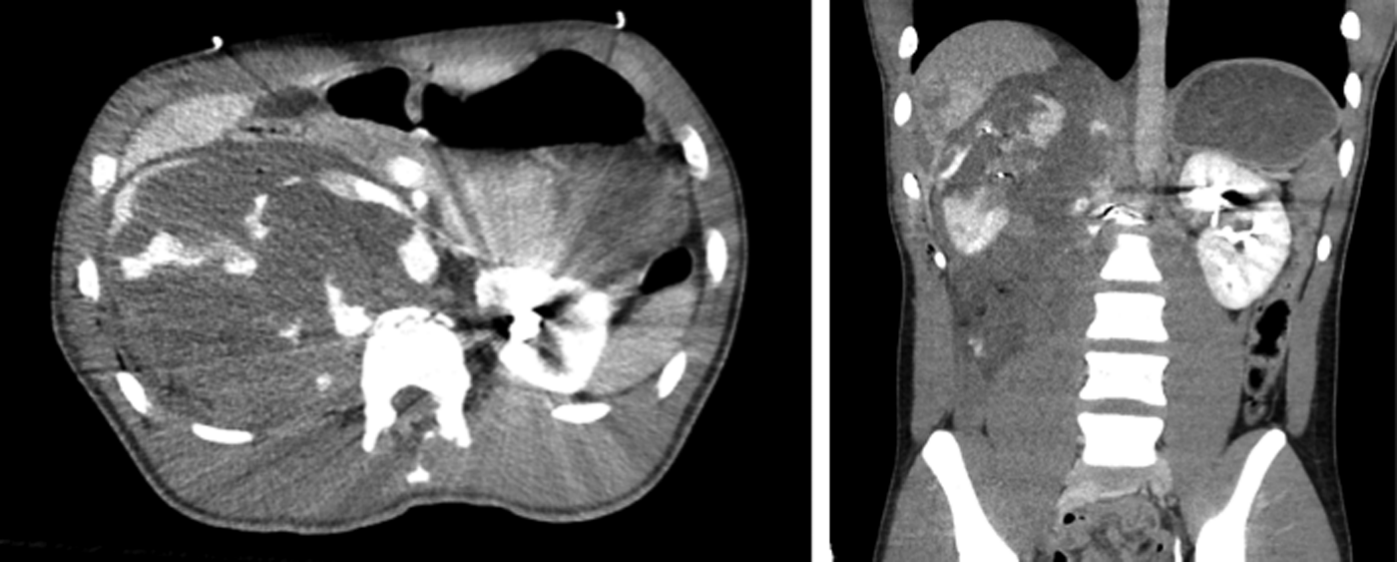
CT abdomen/pelvis showing shattered right kidney and bullet in the upper pole of the left kidney.
